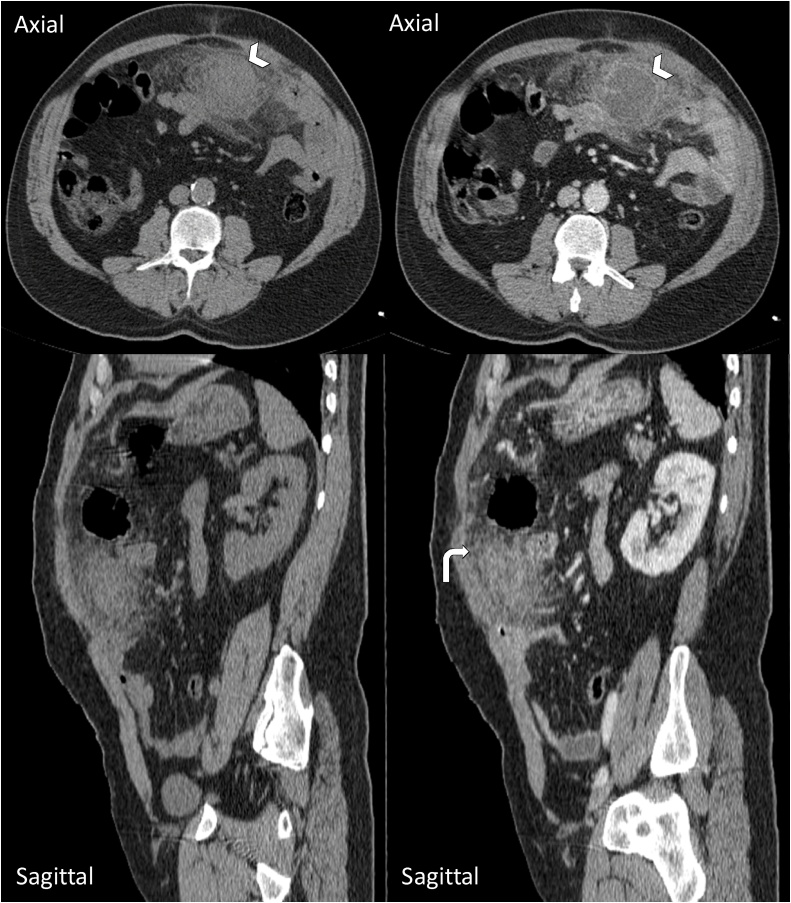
CT Scan images in axial and sagittal views before and after injection of contrast agent showing a supra-umbilical formation (arrowhead), rounded, with low density, and regular wall, enhanced after contrast, containing some fine septa. It is associated with significant local infiltration (curved arrowhead).
